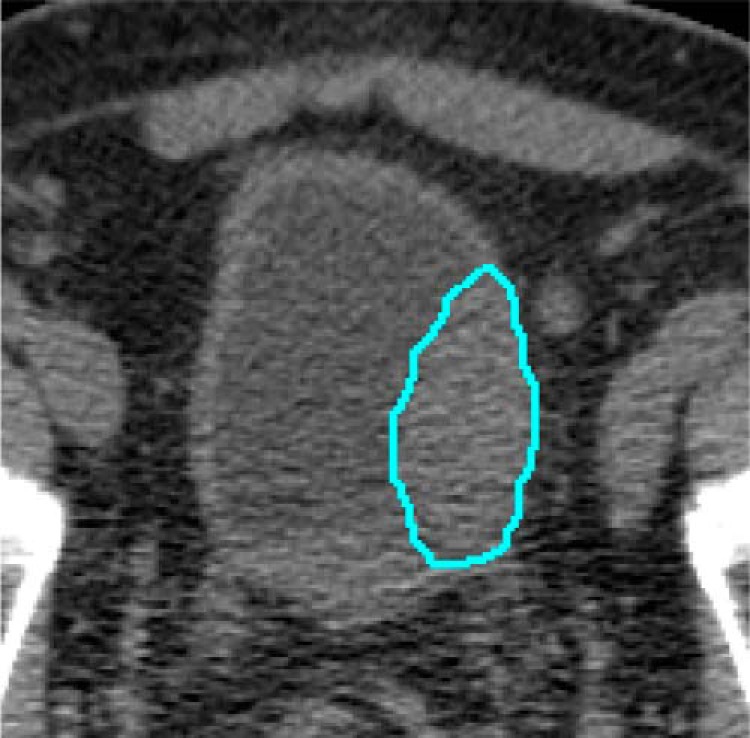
Bladder cancer segmentation on the CT section shown in Figure 1 using the bladder likelihood map shown in Figure 3.
